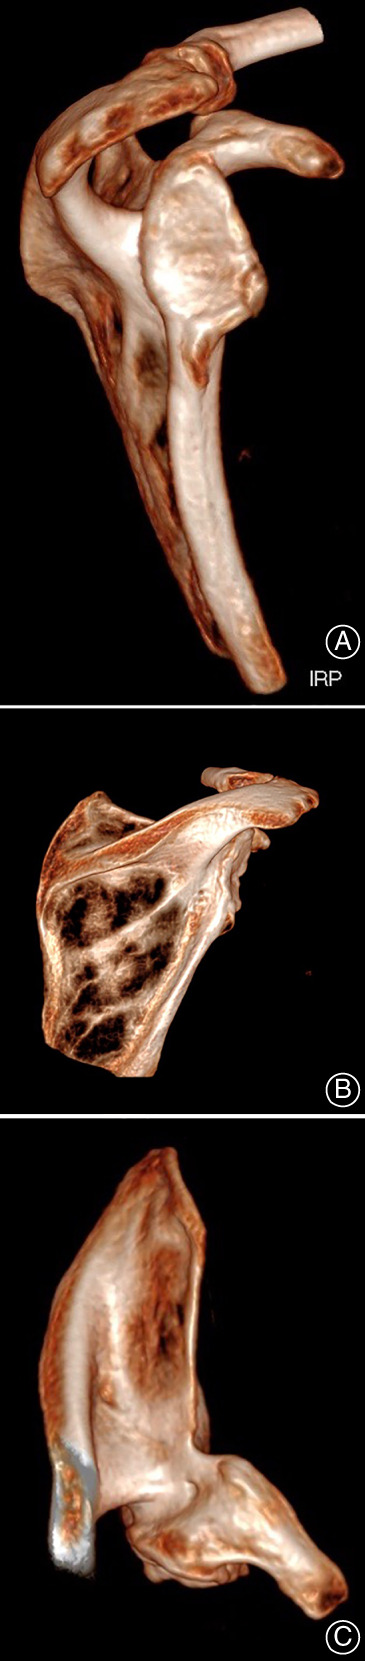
Three‐dimensional CT image of the dysplastic glenoid: (A) lateral (B) posterior, and (C) cranial. The image shows a typical dysplastic glenoid with deficient posteroinferior rim, absence of glenoid neck, retroversion, and inferior inclination of joint surface.
